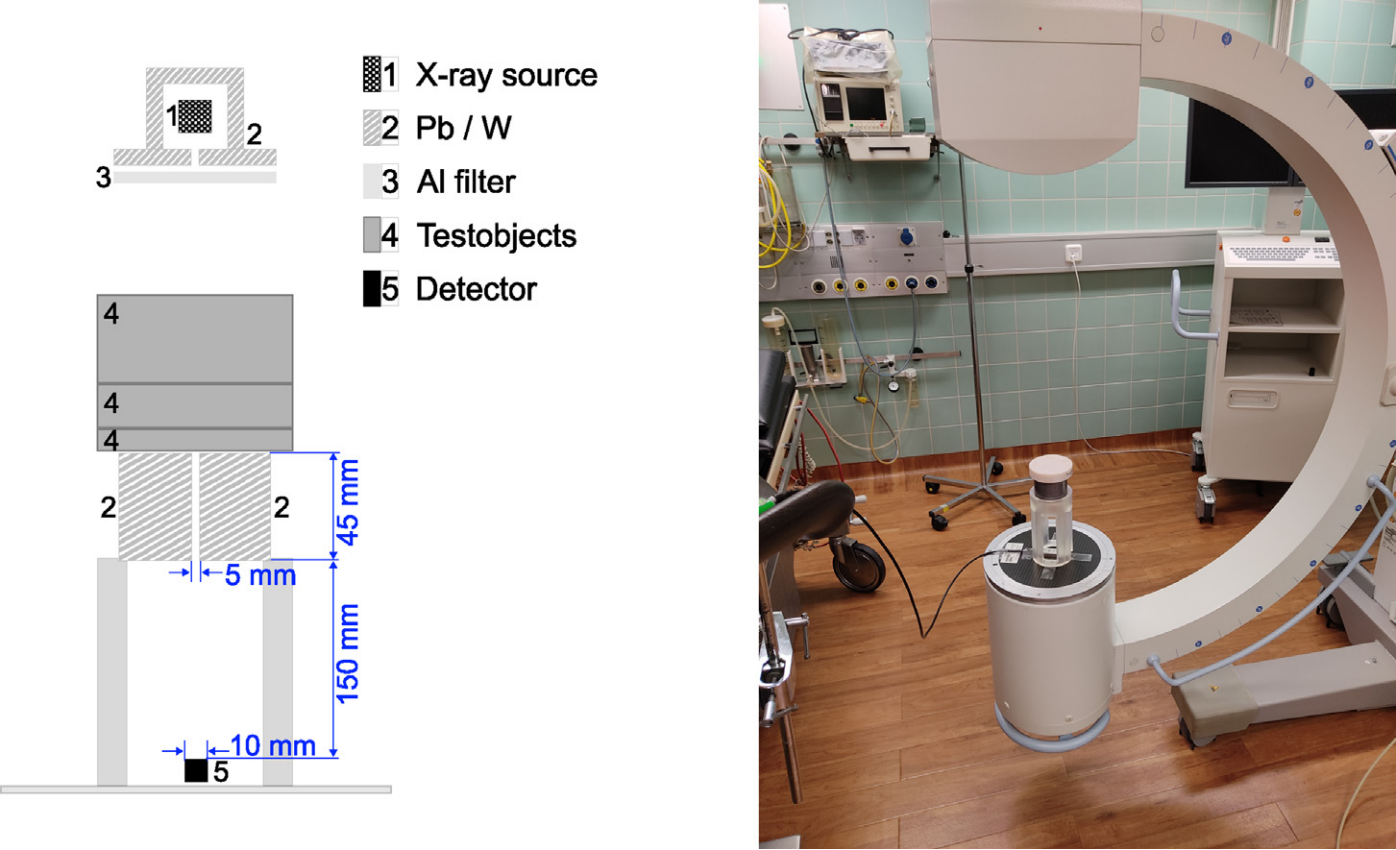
Left: Sketch of the experimental setup in X-ray imaging. Right: Experimental setup with PMMA test object.
